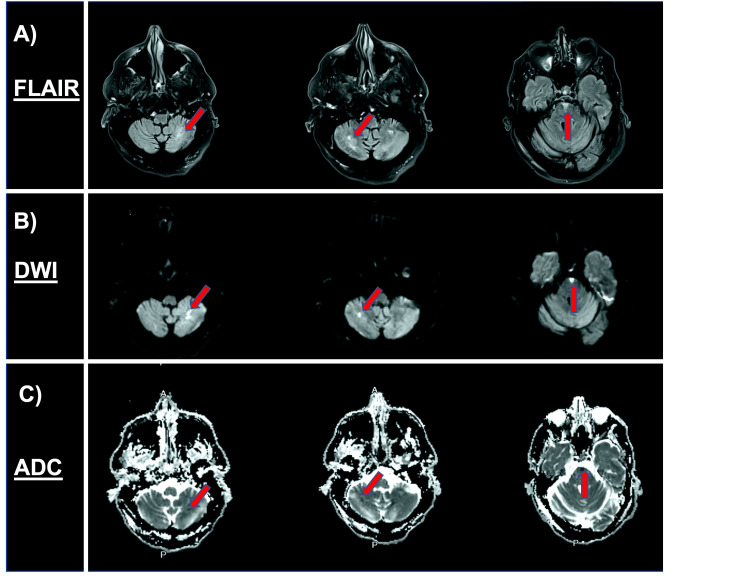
MRI of the brain: acute cerebellar and brainstem infarct FLAIR: fluid-attenuated inversion recovery; DWI: diffusion-weighted imaging; ADC: apparent diffusion coefficient
